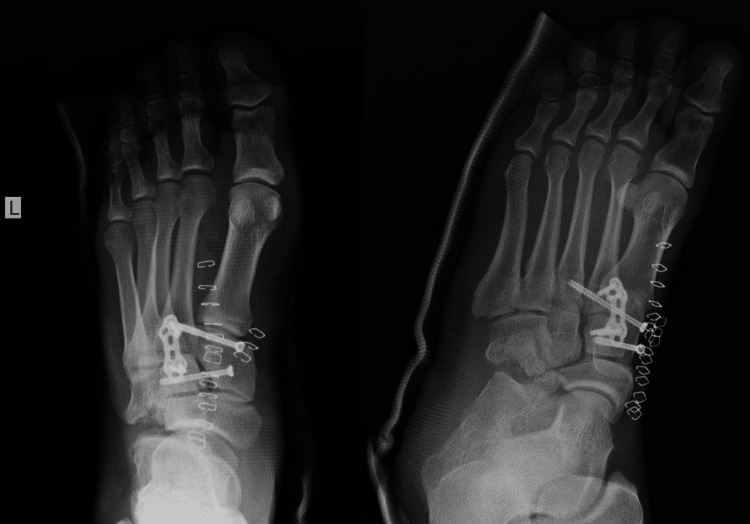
Postoperative foot radiographs
